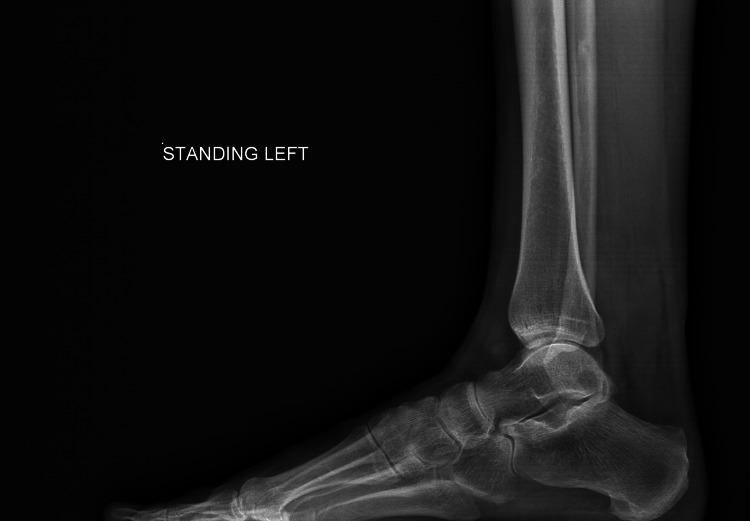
Lateral pre-operative X-ray showing arthritic changes in the left metatarsophalangeal (MTP) joint.
